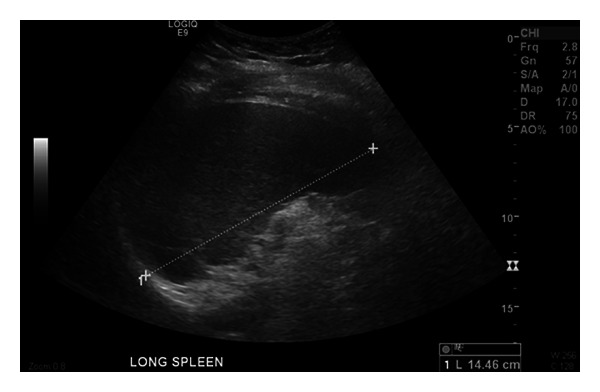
Abdominal ultrasonogram showing the patient's splenomegaly.
